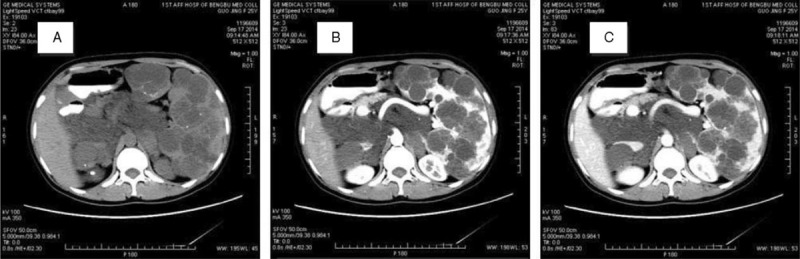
Computed tomography scan demonstrates multiple cystic masses of the abdomen. (A) Plain, (B) arterial phase, (C) delayed phase.
